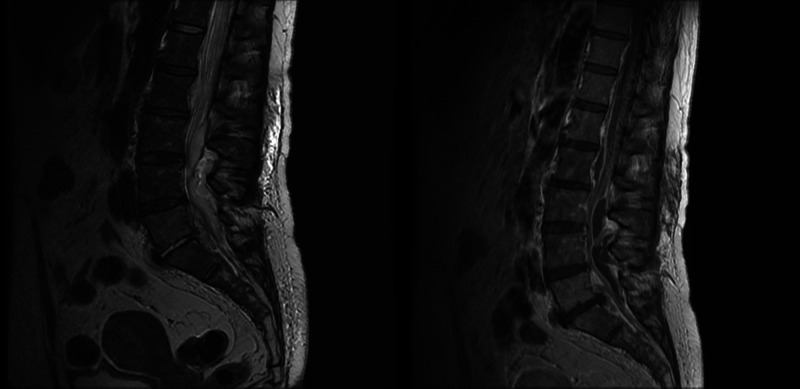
Sagittal T1 and T2-weighted MRI showing an extradural tumor along with the presence of a soft tissue, heterogeneously contrast-enhancing mass, enveloping the roots of the cauda equina, located predominantly at the Th12-L2 level along the anterior and the L3-L5 level on the posterior surface of the dural sac. MRI: magnetic resonance imaging
